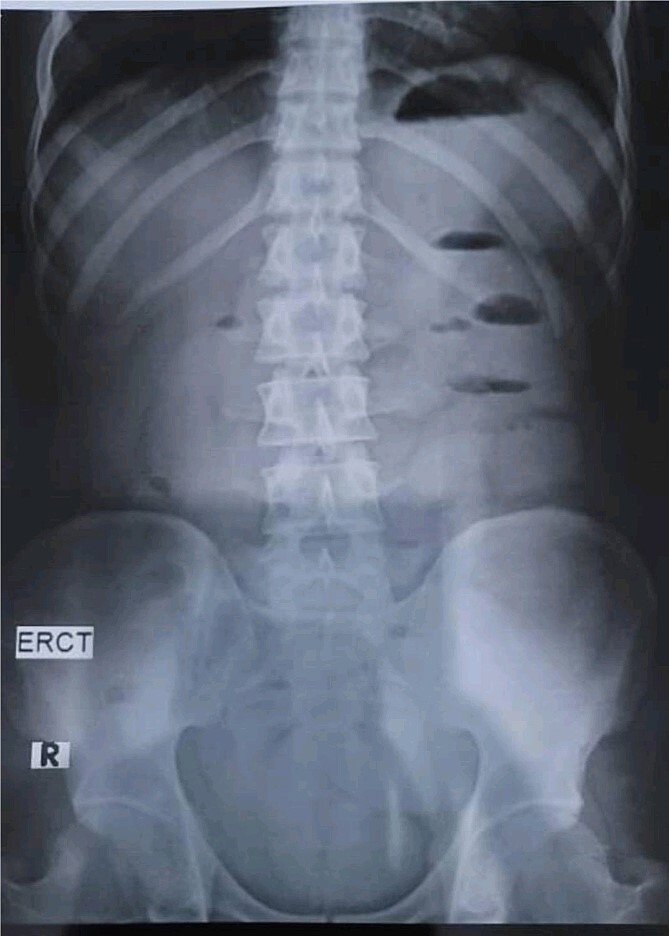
Abdominal X-rays in erect position reveals five air fluid levels.
